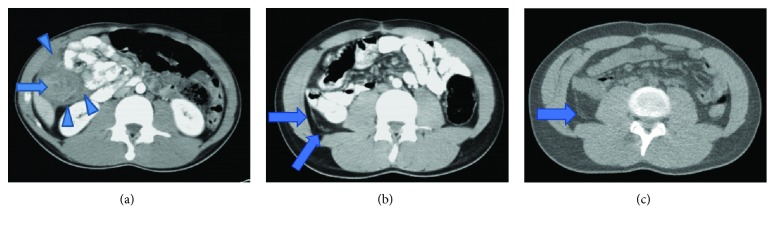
Computed tomography imaging at different stages. (a) Presurgical CT scan: infiltrative mass of the ascending colon causing significant mural thickening and luminal narrowing, associated with minimal surrounding free fluid and peritoneal nodularities. (b) Postsurgical CT scan: minimal residual nodularities at the site of surgery which can represent peritoneal deposits. (c) Postchemotherapy completion CT scan: further decrease and almost complete resolution of the peritoneal nodularities.
